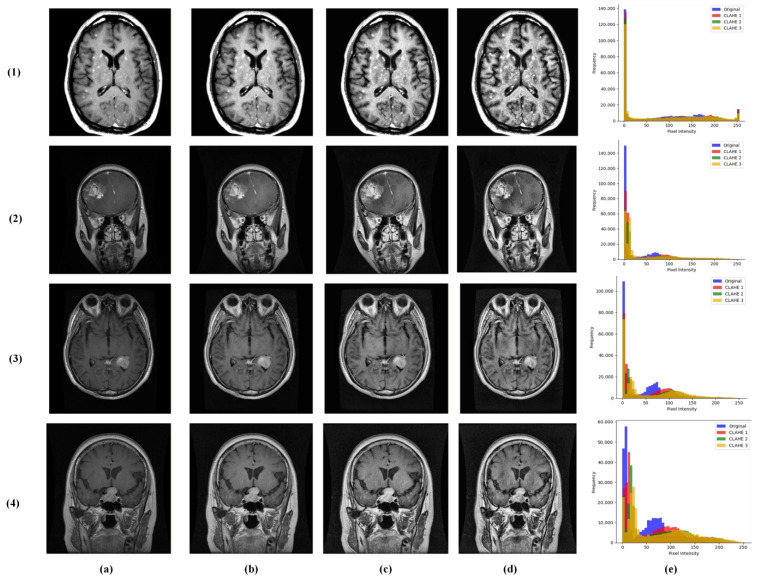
Visualization of multi-contrast CLAHE preprocessing results across the following brain tumor classes: (1) no tumor, (2) glioma, (3) meningioma, and (4) pituitary tumor. Columns represent (a) original input MRI, (b) conservative CLAHE, (c) moderate CLAHE, (d) aggressive CLAHE, and (e) histogram.
